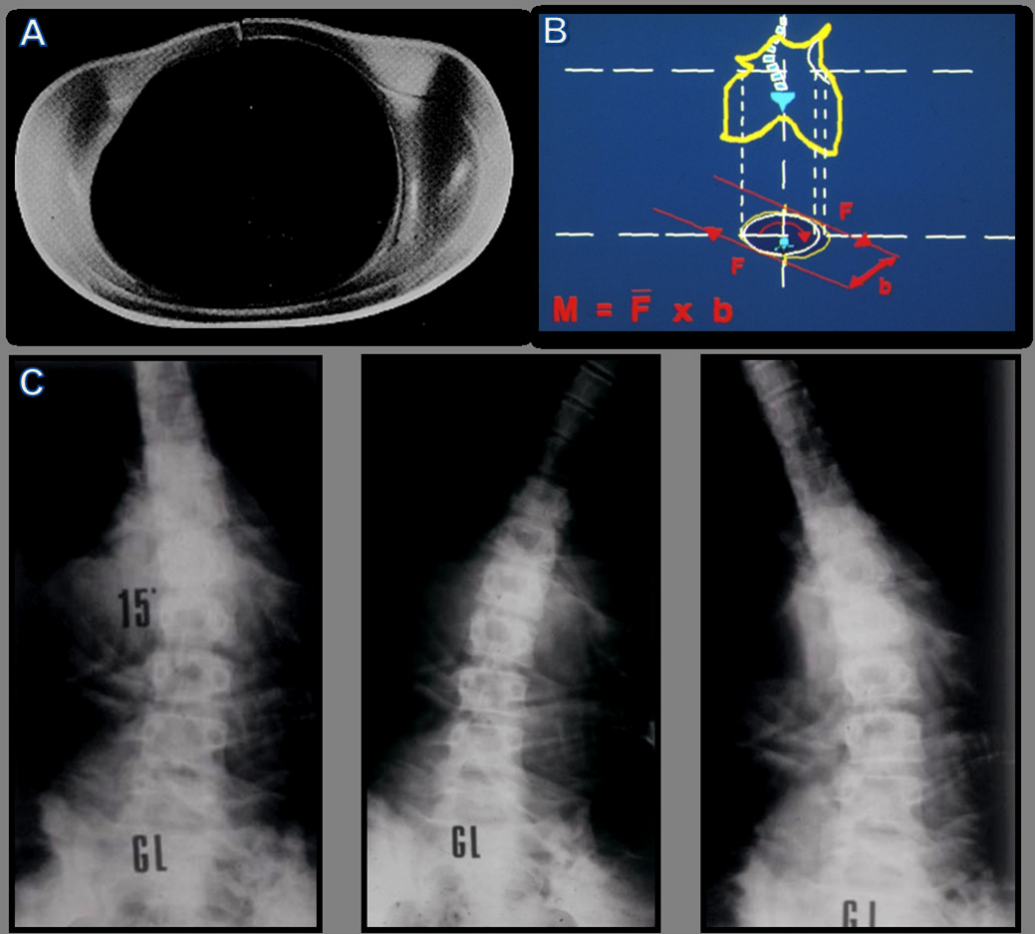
Dynamics constrained by the brace geometry. The trasverse section of the brace (A), elliptical and asymmetrical up to the plan of the pelvic hold, produces twisting moments opposed to the direction of rotation of the vertebrae included in the curve (b). The bending X-ray (c) shows the efficacy of the twisting action produced by the brace in all movements of the trunk.
